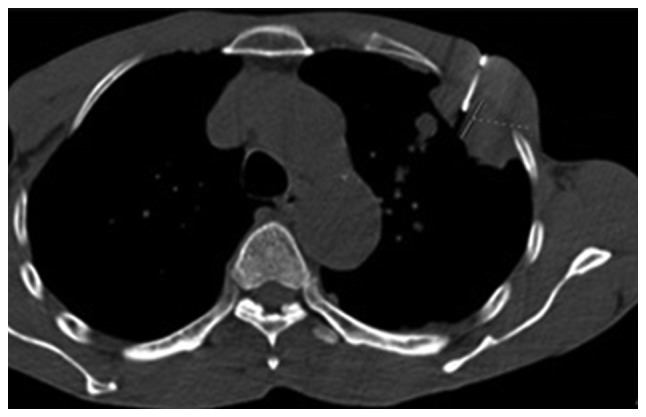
Male, aged 75 years, the result of the computed tomography-guided cutting needle pleural biopsy was malignant mesothelioma.
